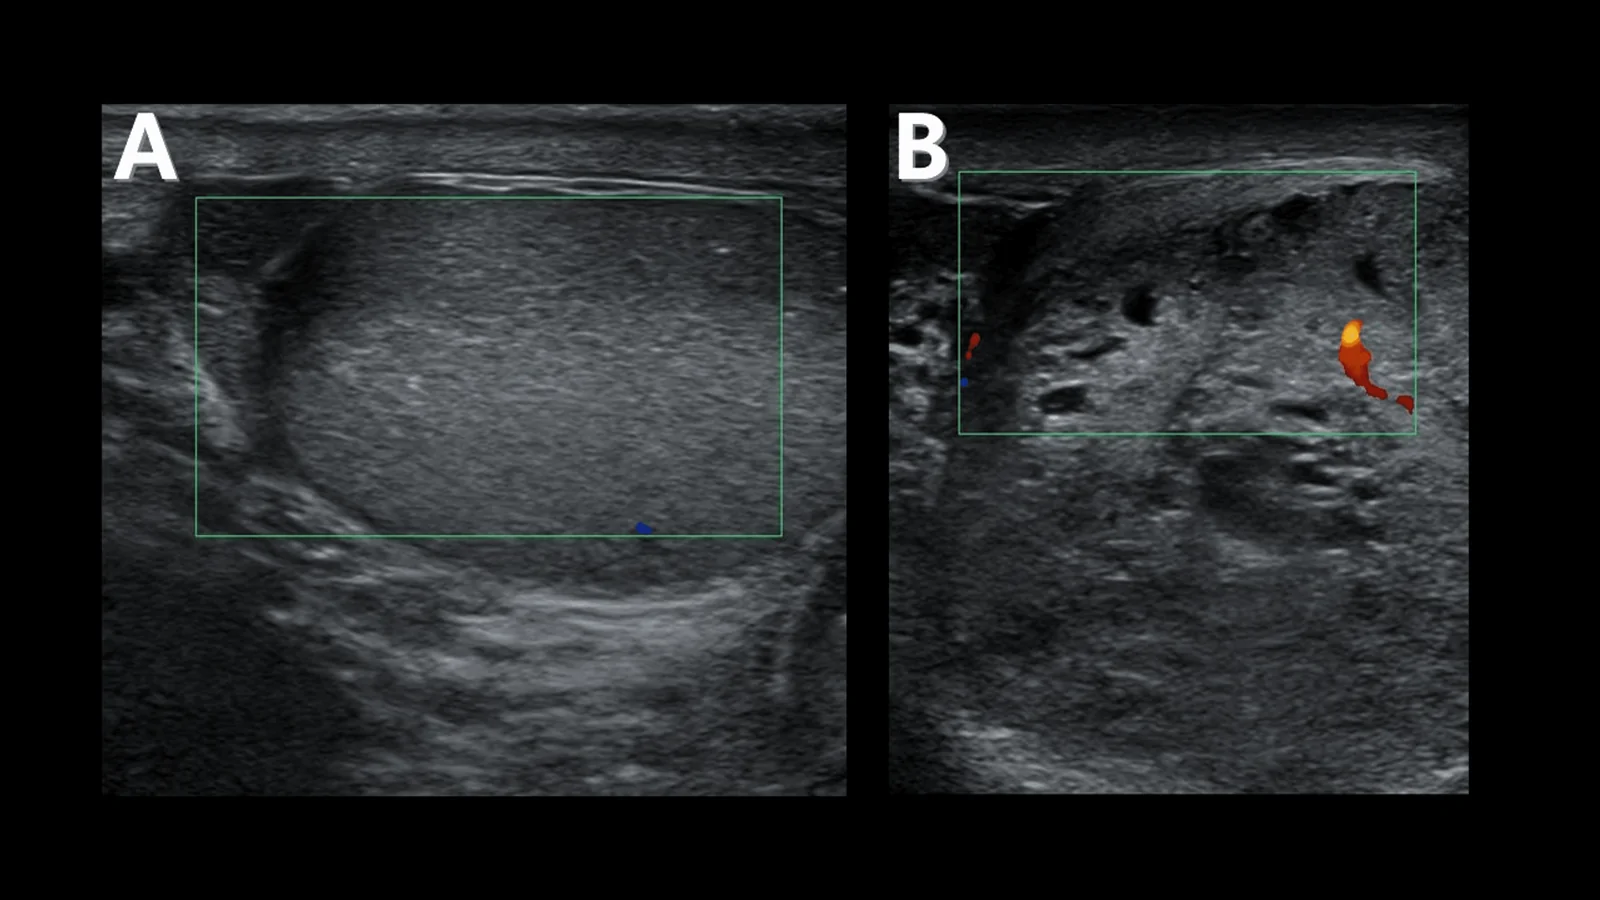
Color Doppler ultrasonography of the testis (A) Color Doppler sonography of the right testicle demonstrates a solid, heterogeneous intratesticular mass with minimal internal vascular flow, reflecting extensive central necrosis. (B) Additional imaging reveals multiple cystic spaces within the intratesticular mass, demonstrating a hypervascular pattern characteristic of viable tumor components
